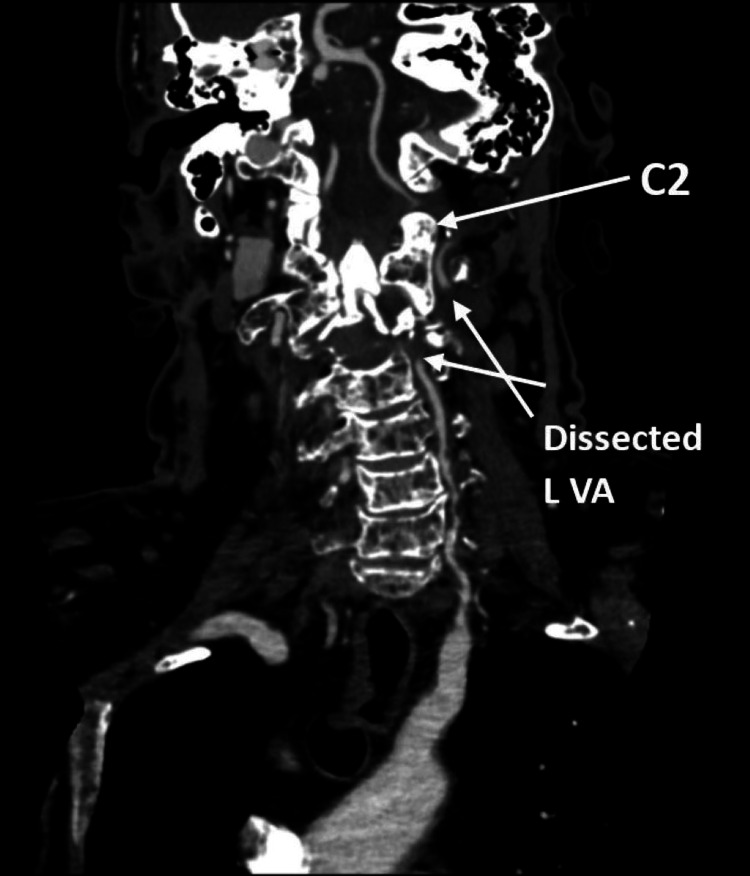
CT angiogram displaying an expansile lytic lesion of the C2 vertebral body and moderate, focal compression of the distal right V2 segment with severe stenosis of the proximal left V2.
